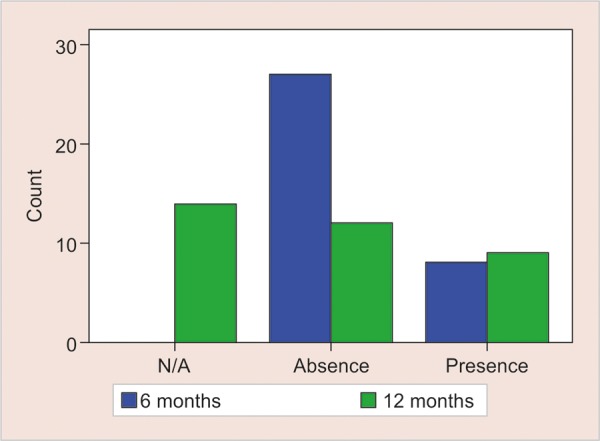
Status of radicular pulp lumen thinning results from radiographic follow- ups at 6 and 12 months
